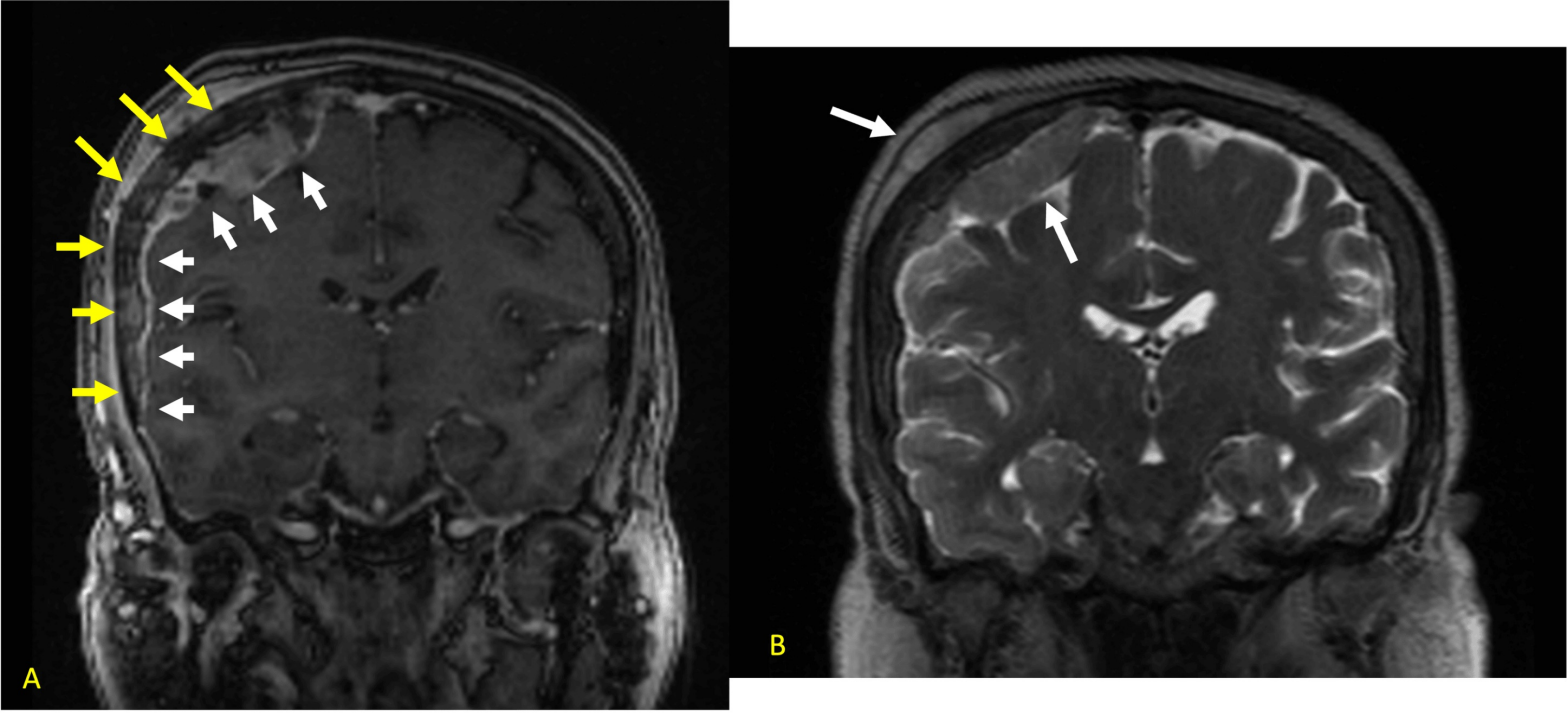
a. Coronal T1 MRI brain image demonstrating epidural disease with a long segment of dural infiltration, skull invasion, and a large extracranial component elevating the skin. b. Coronal T2 MRI demonstrating brain displacement without cerebral edema.
